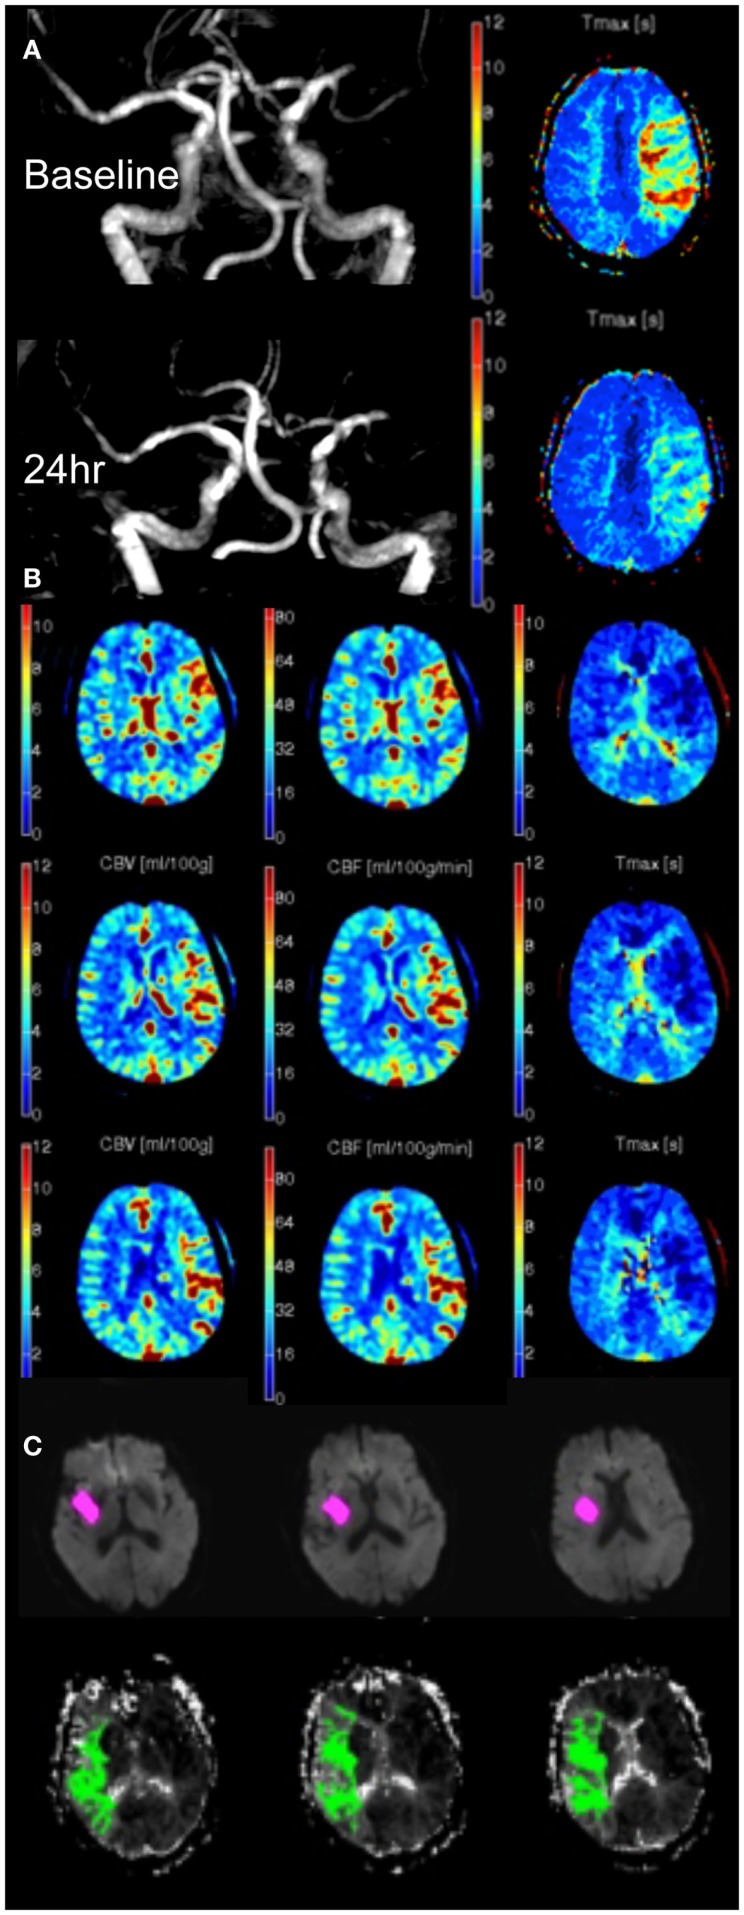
(A) MRA and *T*_max_ in a patient with left MCA occlusion at 5 and 24 h. Despite persistent occlusion, the volume of *T*_max_ > 6 s has reduced from 56 to 0 mL due to improved collateral supply. (B) Post-reperfusion hyperperfusion indicated by increased CBV (left), CBF (middle), and reduced *T*_max_ (right) in a patient with recent reperfusion of left MCA occlusion. (C) MRI diffusion (pink) and perfusion (*T*_max_ > 6 s, green) imaging 24 h post-stroke onset showing persistent hypoperfused tissue, which was contributing to the patient’s clinical deficit but had not developed diffusion restriction.
